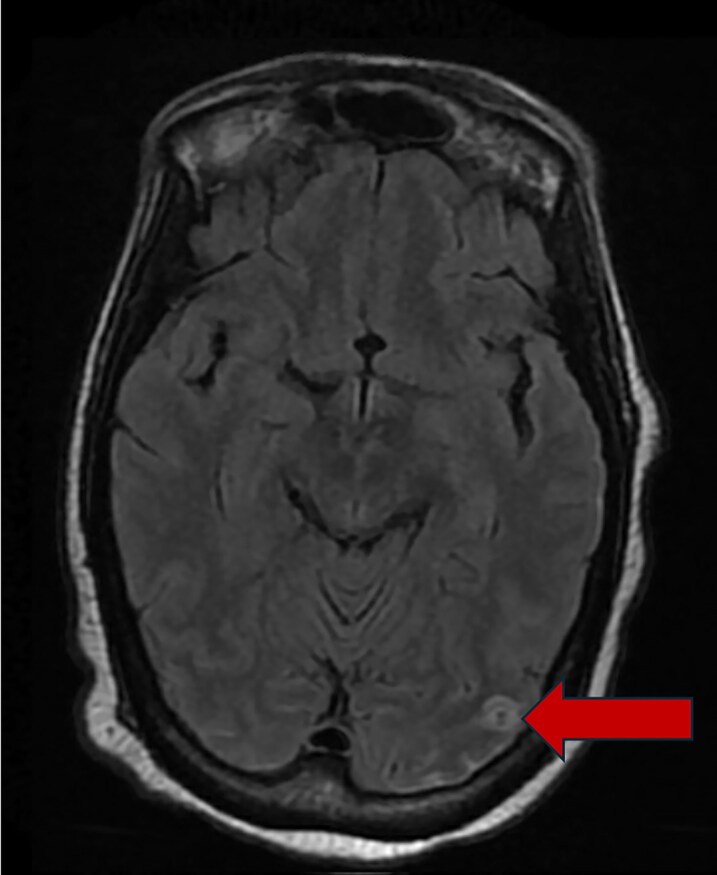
MRI brain showing left occipital lesion.
